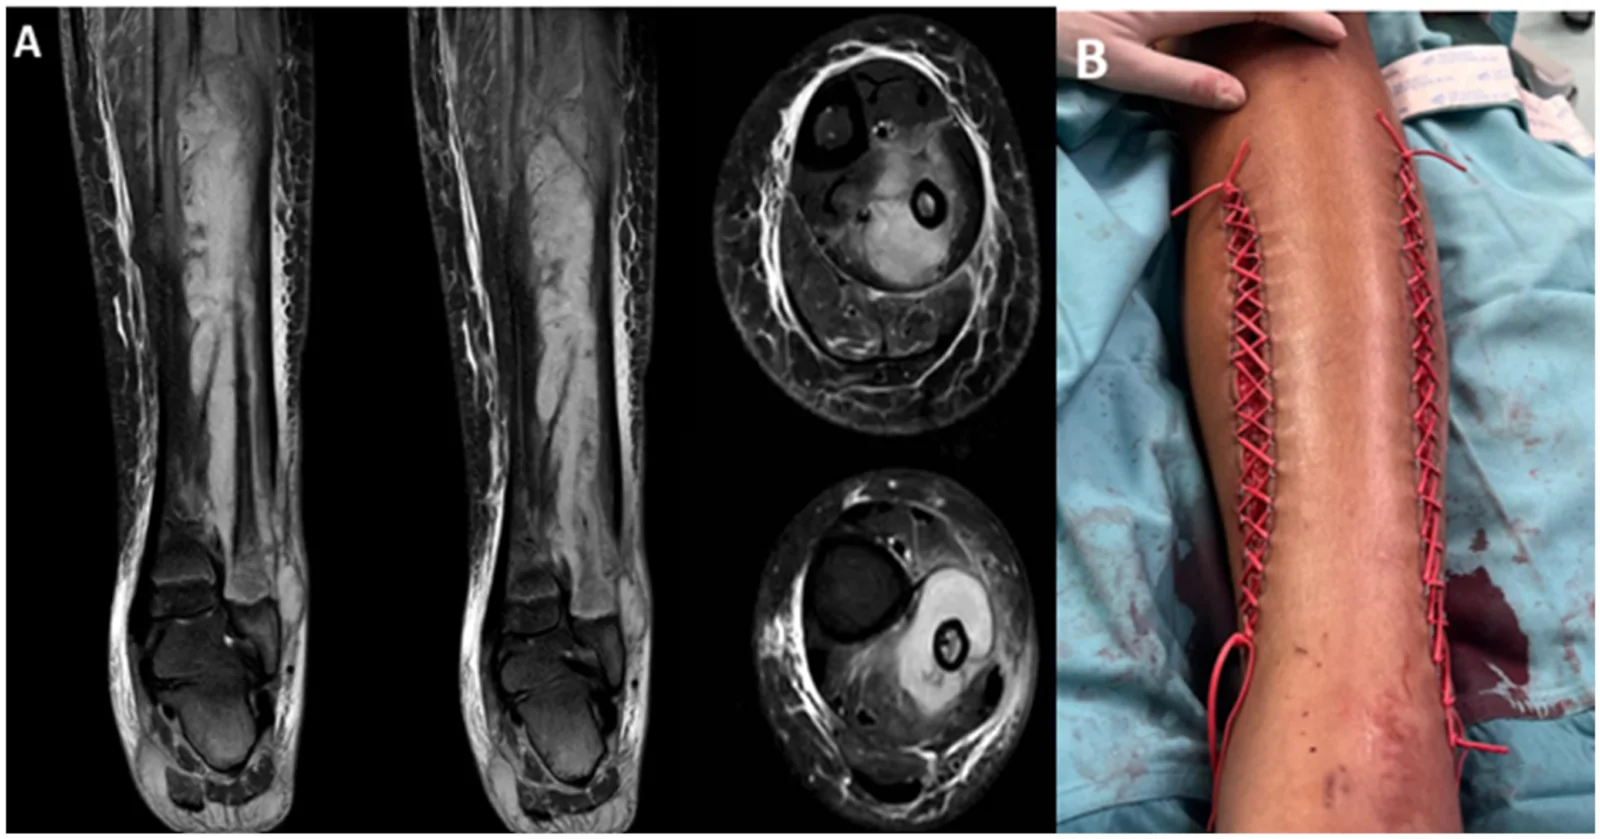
A 12-year-old patient with a history of a previous ankle sprain. MRI demonstrates peroneal osteomyelitis with a large abscess collection in the deep posterior compartment, accompanied by superficial and deep fasciitis (A). Post-operative outcome following compartment decompression and debridement via medial and lateral leg incisions, with skin closure achieved using the shoelace technique (B).
